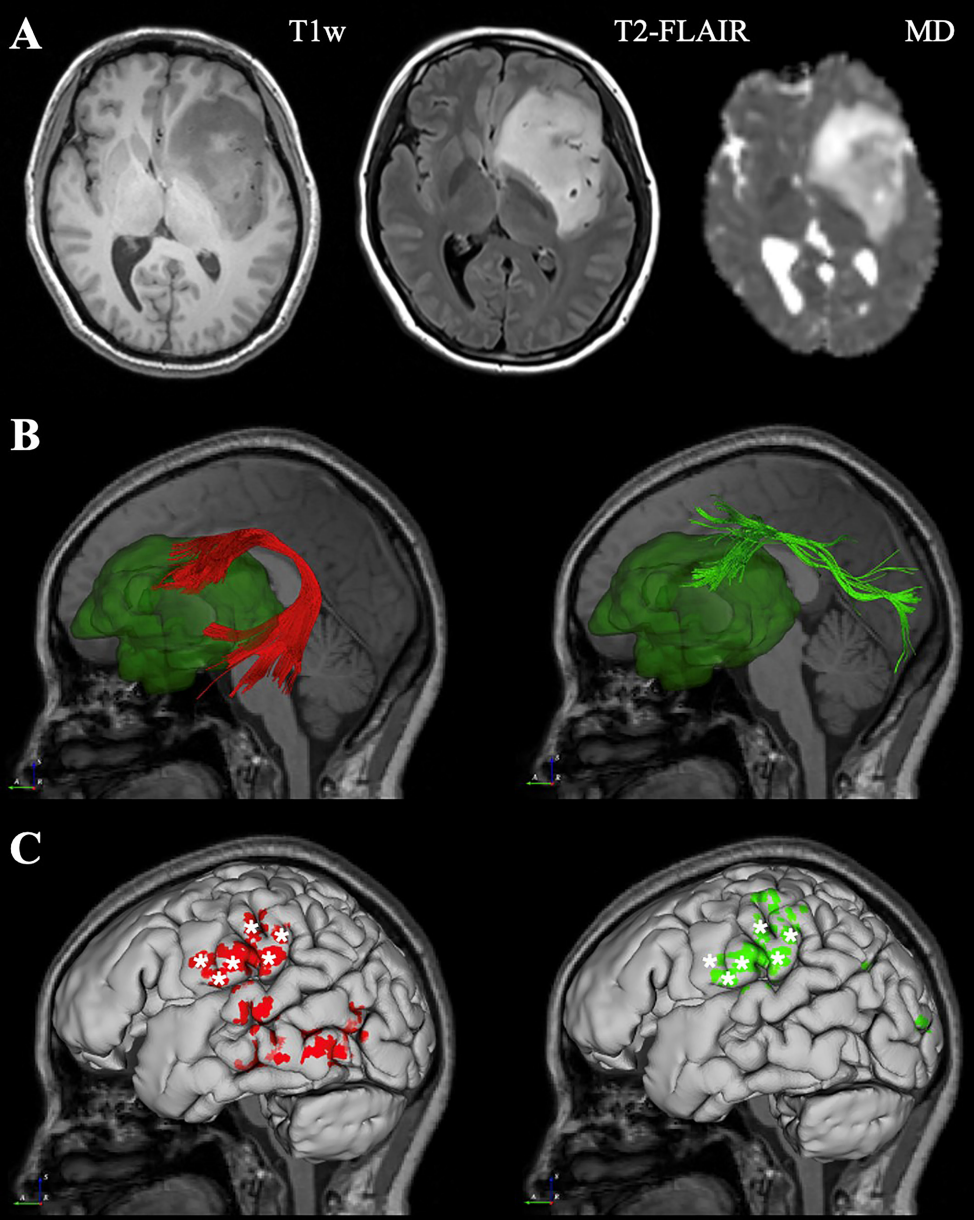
Representive imaging of a patient with left frontal-temporal lobe astrocytoma. Case 1, a 42-year-old woman with left frontal-temporal lobe astrocytoma (WHO grade II) in MR images (A). The relationship between language-related fiber tracts (AF in red and SLF-II in green color) and tumor (dark green color) were shown in (B). The cortical termination of each tract was projected on the cortical surface (C). The eloquent area of intro-operative speech arrest (DCS) was marked with stars. (MD, Mean Diffusivity).
